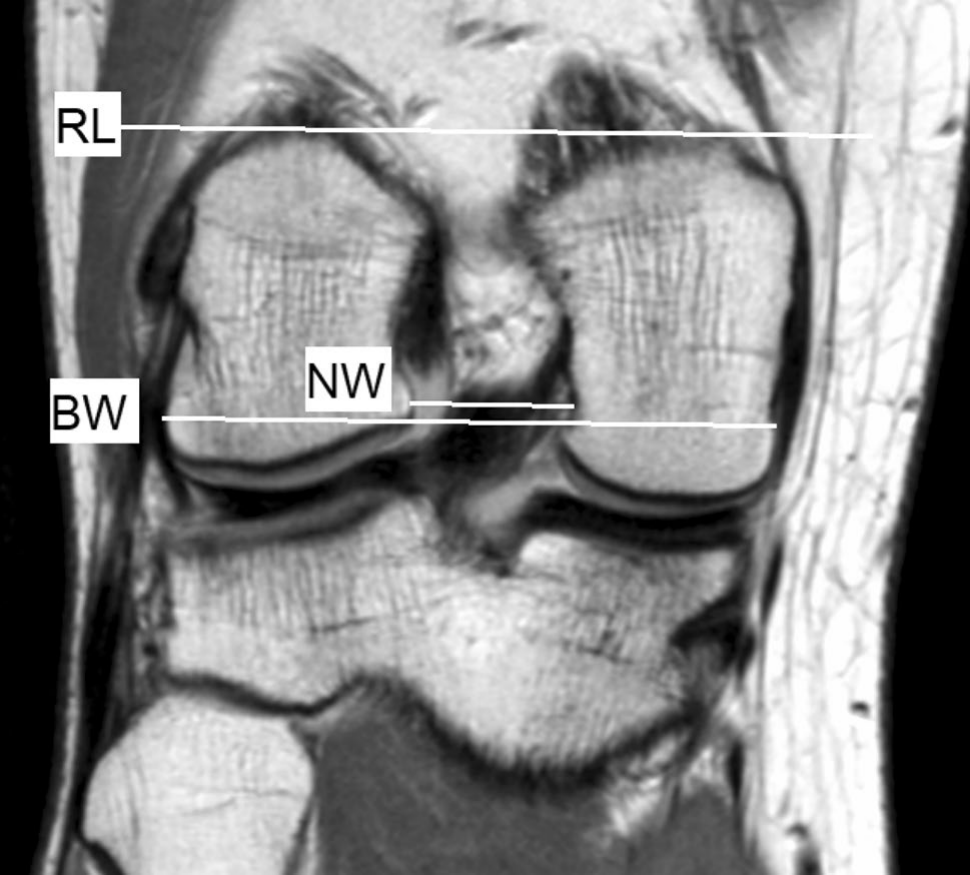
Coronal MRI of the knee, with the described measurements. *RL* reference line, *NW* notch width, *BW* bicondylar width
